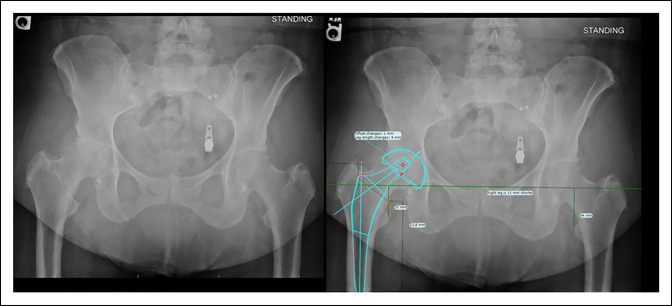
Template AP pelvic radiograph shows superior migration of the femoral head in (A), and the template in (B) shows the plan to restore the leg length and offset.
